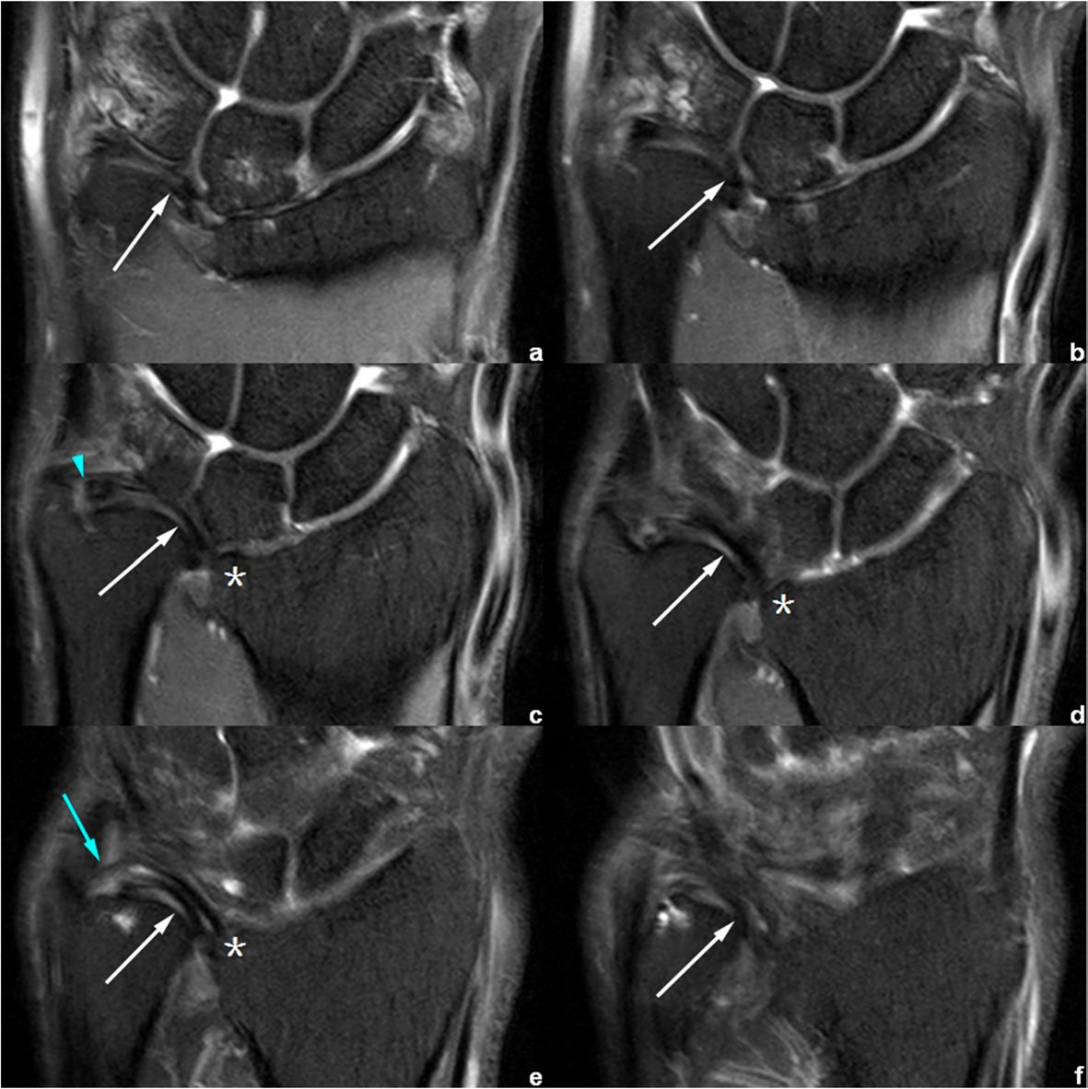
Right wrist MRI of the patient No. 0756. a–f Coronal PDWI-FS images show that the CD (white arrow) inserted at the distal articular surface level (*) of radius, and attched to distal ulna via distal (blue arrowhead) and proximal (blue arrow) triangular ligaments. Notice that the CD had degenerative changes due to the abnormal stress. This adult patient had manifestation similar to Madelung’s deformity, but without VL and/or RTL developed. He was diagnosed with Madelung-like deformity of right wrist, according to imaging fingdings and medical history
